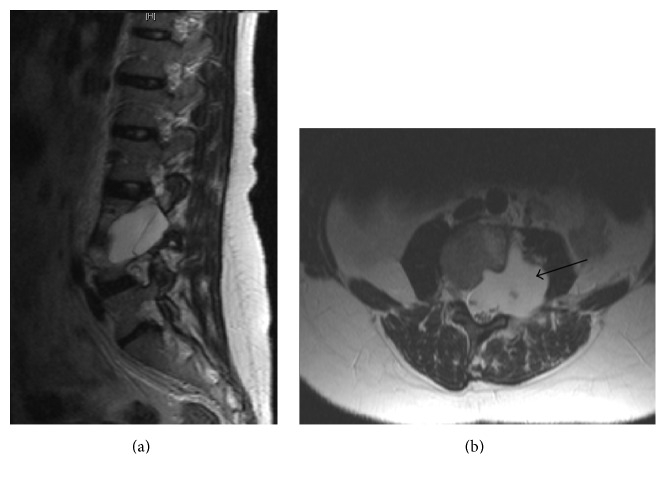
Preoperative MRI sagittal and axial T2-weighted postgadolinium images showed a pseudomeningocele at L3-L4 levels with paravertebral extension and psoas muscle remodeling (black arrow).
